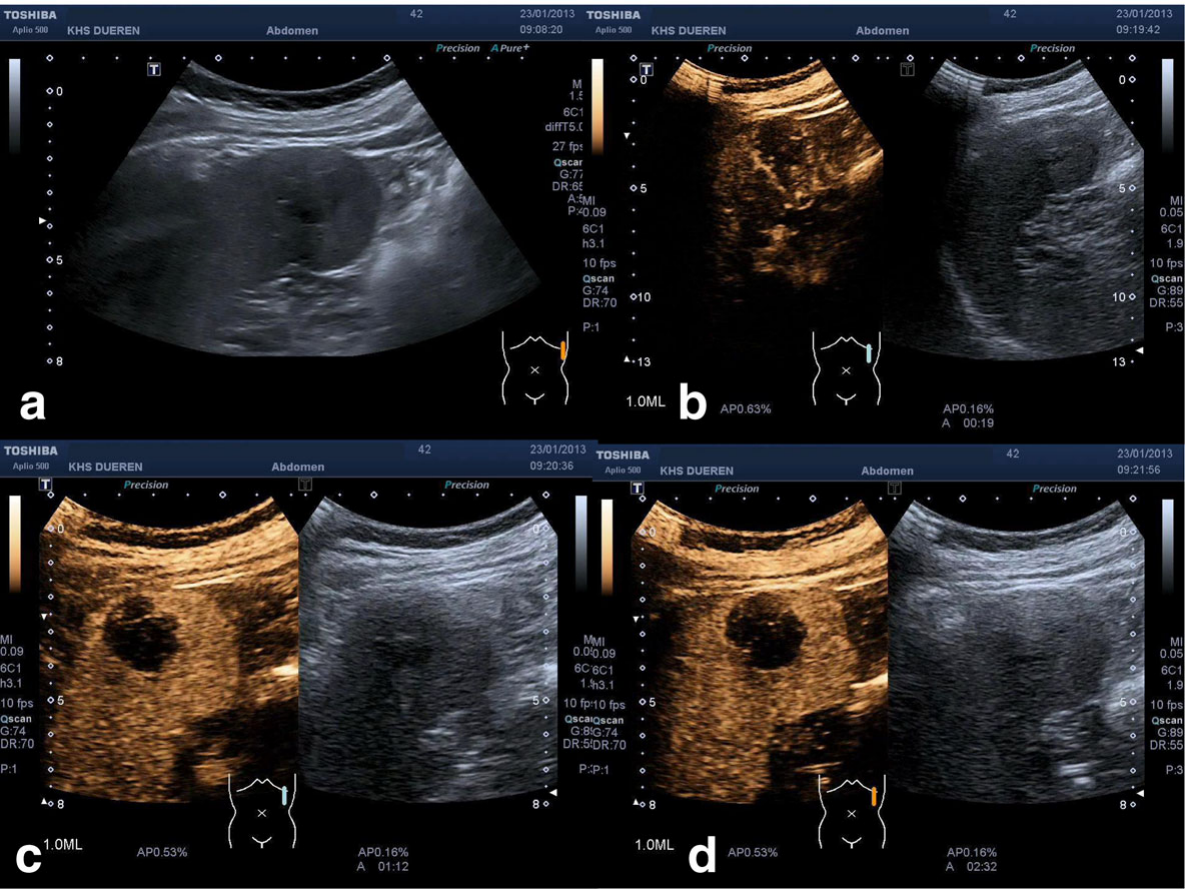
a B-mode ultrasound picture of the lesion. b, c, d Contrast-enhanced ultrasound image 19, 72, and 152 seconds after injection of 1 ml SonoVue®
